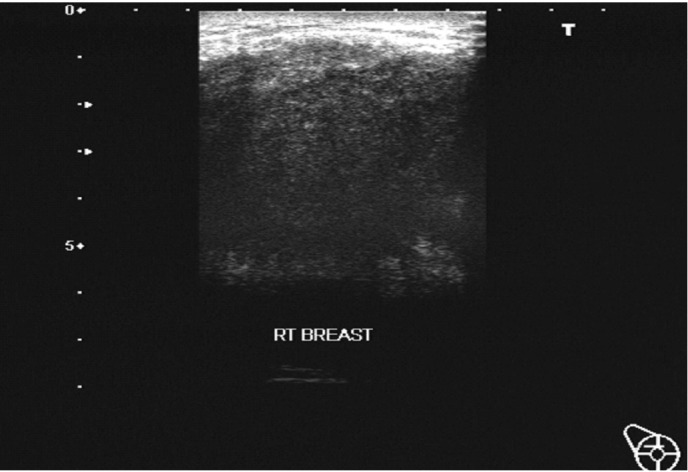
A 26-year-old transgender with primary breast lymphoma. Ultrasound of the right breast shows a large irregular predominant hypo-echoic mass with heterogeneous internal echotexture and cast posterior shadow.
